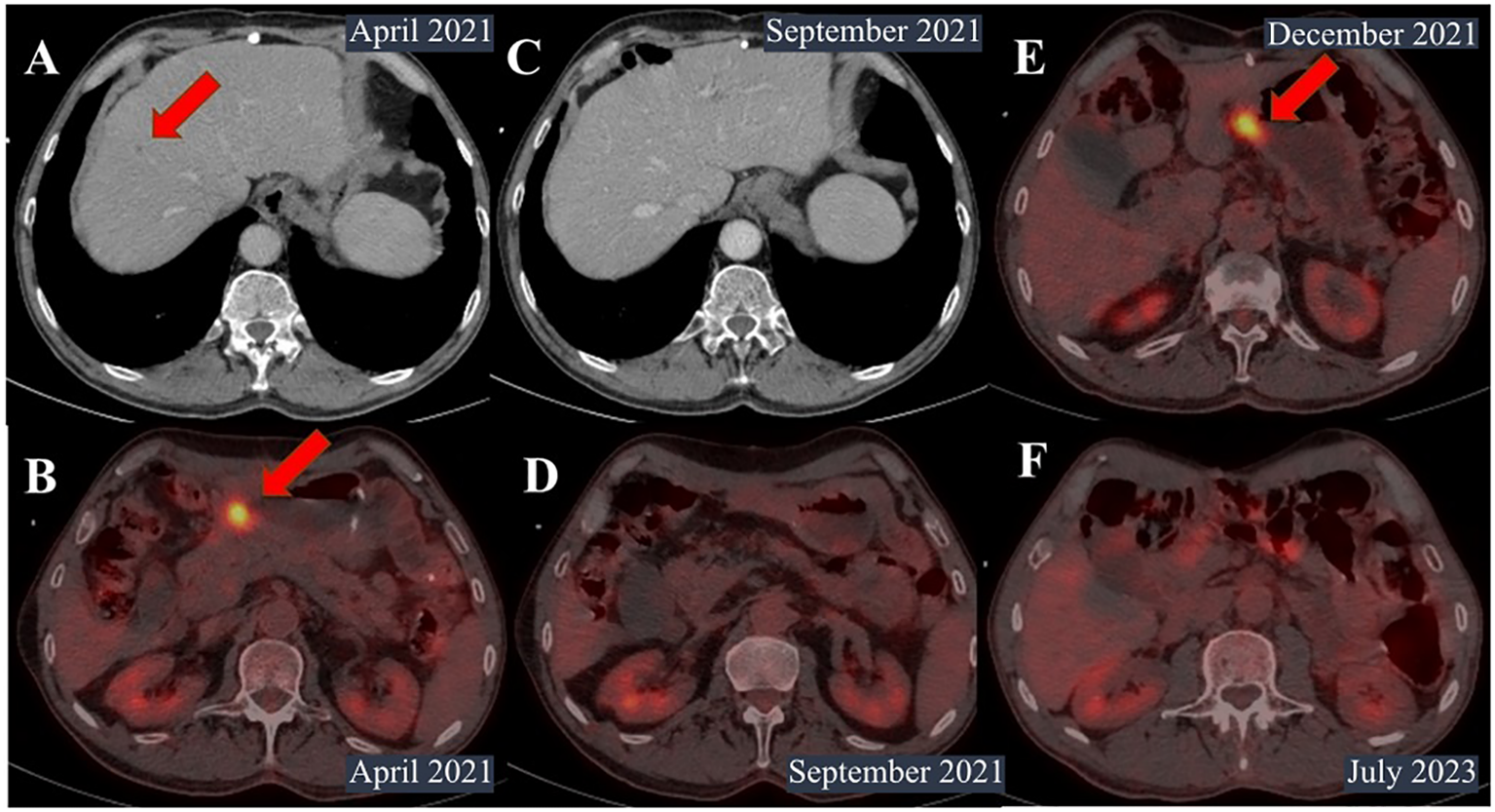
Contrast-enhanced computed tomography (CT) and PET scan images. (A) CT scan in April 2021. The size of metastatic foci in the S8 segment of the liver decreased from 11 mm to 8 mm. (B) Positron emission tomography (PET) in April 2021 showed a decrease in the size of infiltration in the distal part of the stomach from 73 mm × 104 mm to 39 mm × 28 mm. (C, D) PET scan in September 2021. No metastases in the liver or lymph nodes were identified. (E) PET scan in December 2021. The primary tumor was noted in the small curvature of the pylorus up to 18 mm × 24 mm with hypermetabolism of fluorodeoxyglucose (SUVmax=11.29). (F) PET scan in July 2023. No signs of recurrence were noted.
